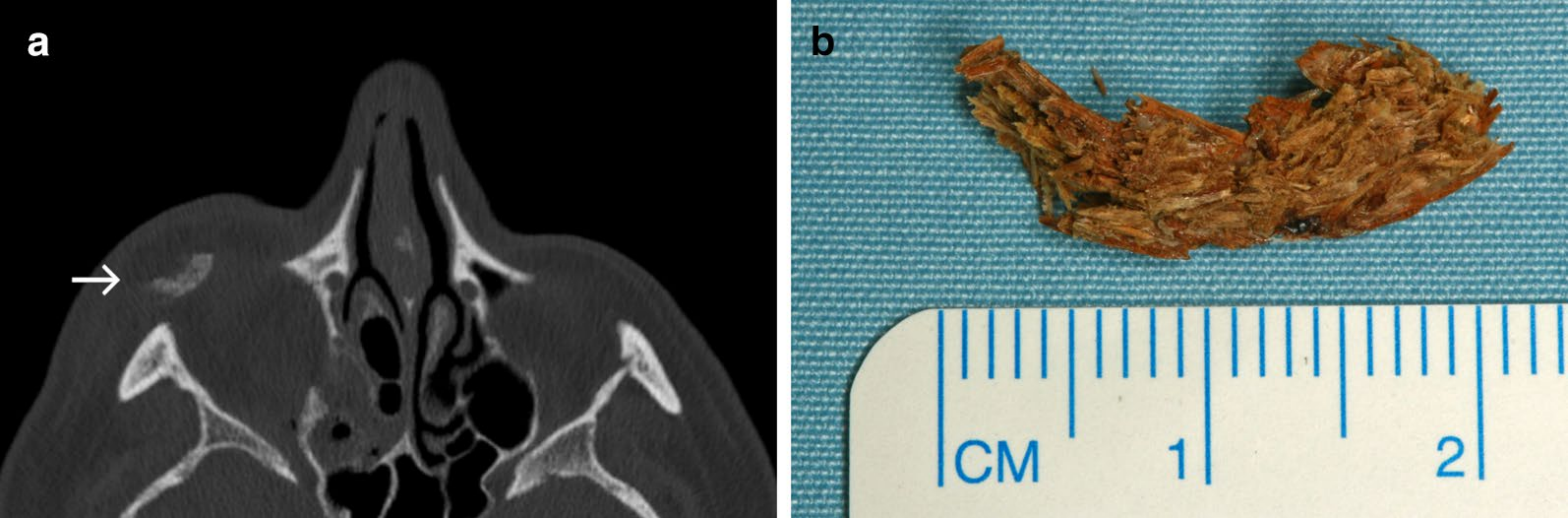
Chipboard wood. a An axial CT image displaying a hyperdense structure dislocated in periorbital soft tissues inferior and anterior to the right globe (arrow). Hyperdensity of the foreign object in this case is likely due to its industrial processing. b A photograph of a chipboard fragment after its removal from the soft tissues
